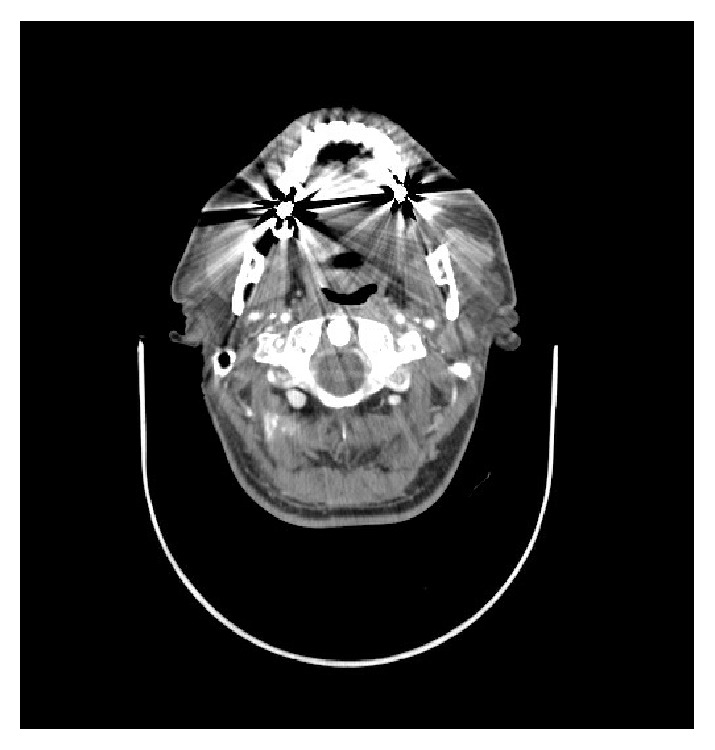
CT scan with contrast axial view. Despite severe metal streak artefacts due to presence of amalgam, a homogenous enhancing lesion measuring about 17*∗*15 mm is demonstrated in the deep portion of left parotid gland.
